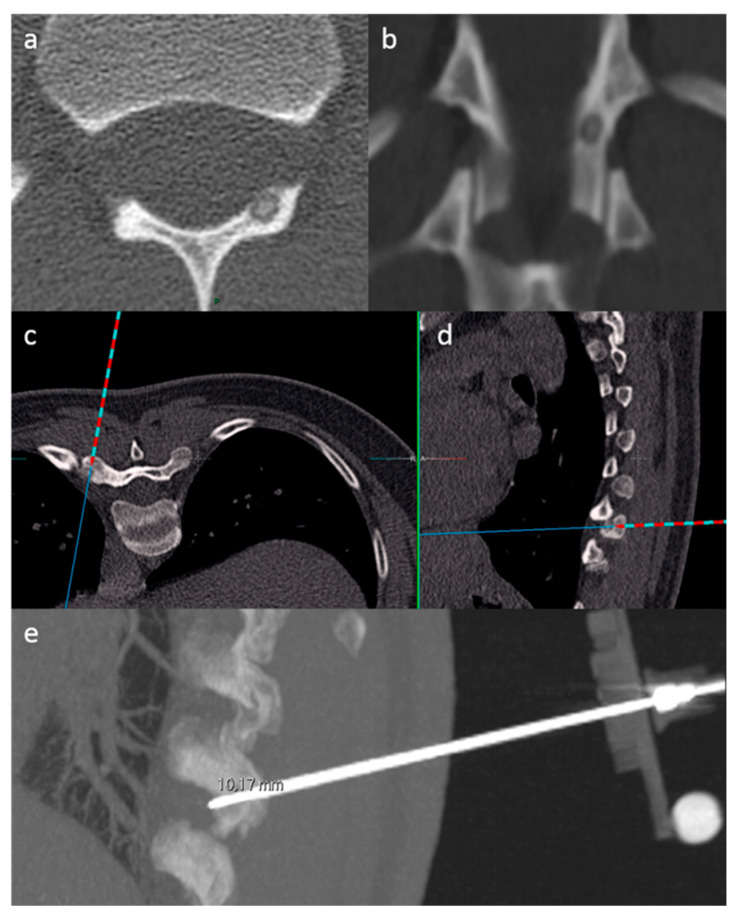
SIRIO-assisted needle positioning within an osteoid osteoma of the left lamina of a thoracic vertebra, in order to perform a biopsy procedure. Figures in (a,b) show CT images of the osteoid osteoma. Axial (c) and sagittal (d) reconstructions of the virtual 3D model show the needle trajectory to reach the lesion. The sagittal reconstruction in (e) confirms the correct positioning of the needle within the lesion; notice the needle tool with a passive sphere included in the CT scan (lower right).
